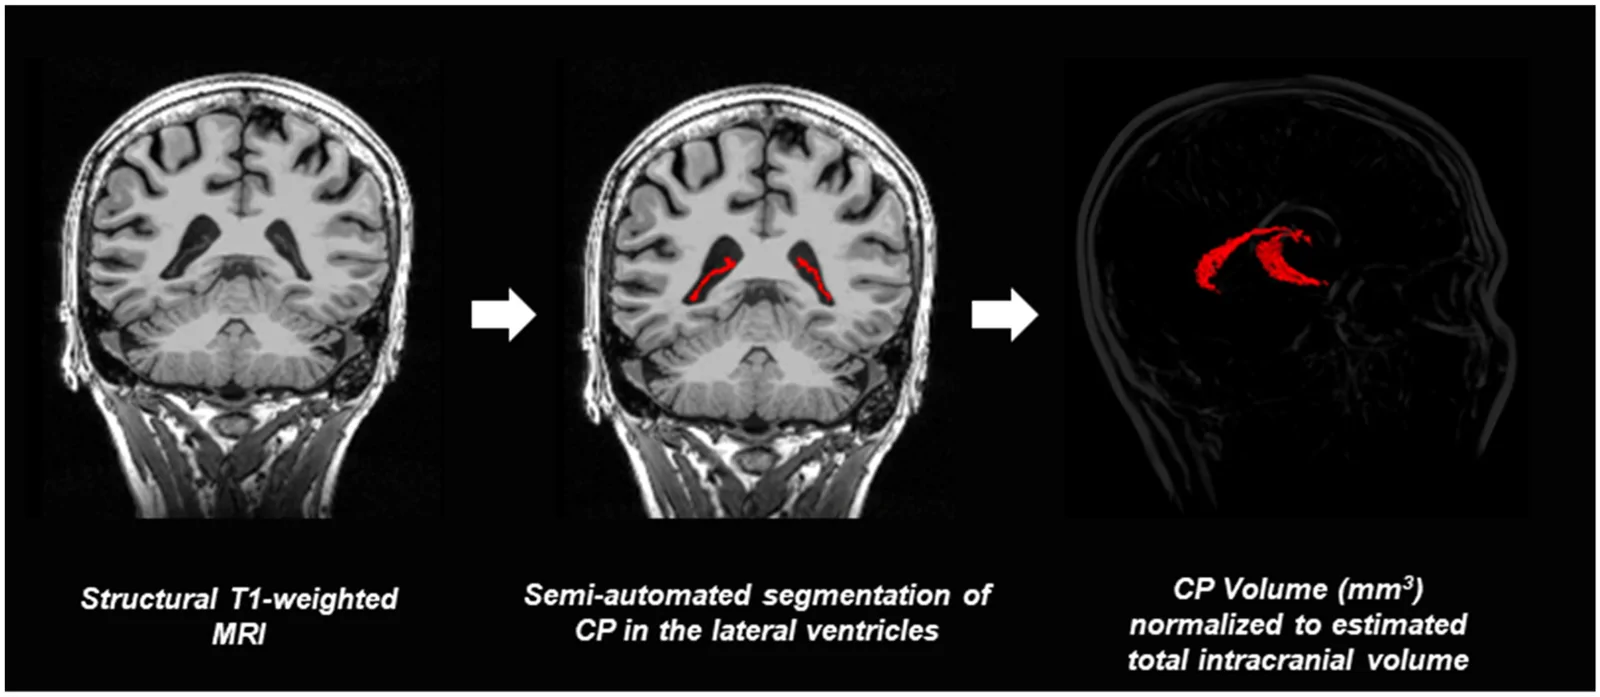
Semi-Automated Segmentation of the Choroid Plexus from T1-weighted images. A Gaussian Mixture Model [20] was used to automatically parcellate the CP within the lateral ventricles (using FreeSurfer-derived masks). The resulting segmentation was then manually corrected using ITK-SNAP [41] and normalized to the FreeSurfer-estimated total intracranial volume. Magnetic Resonance Imaging (MRI), Choroid Plexus (CP), Insight Segmentation and Registration Toolkit (ITK).
